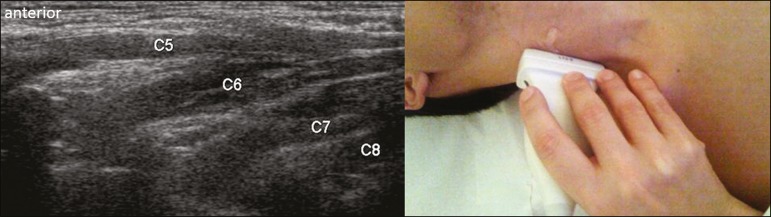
Ultrasound in the longitudinal plane, showing the C5-C8 roots.
